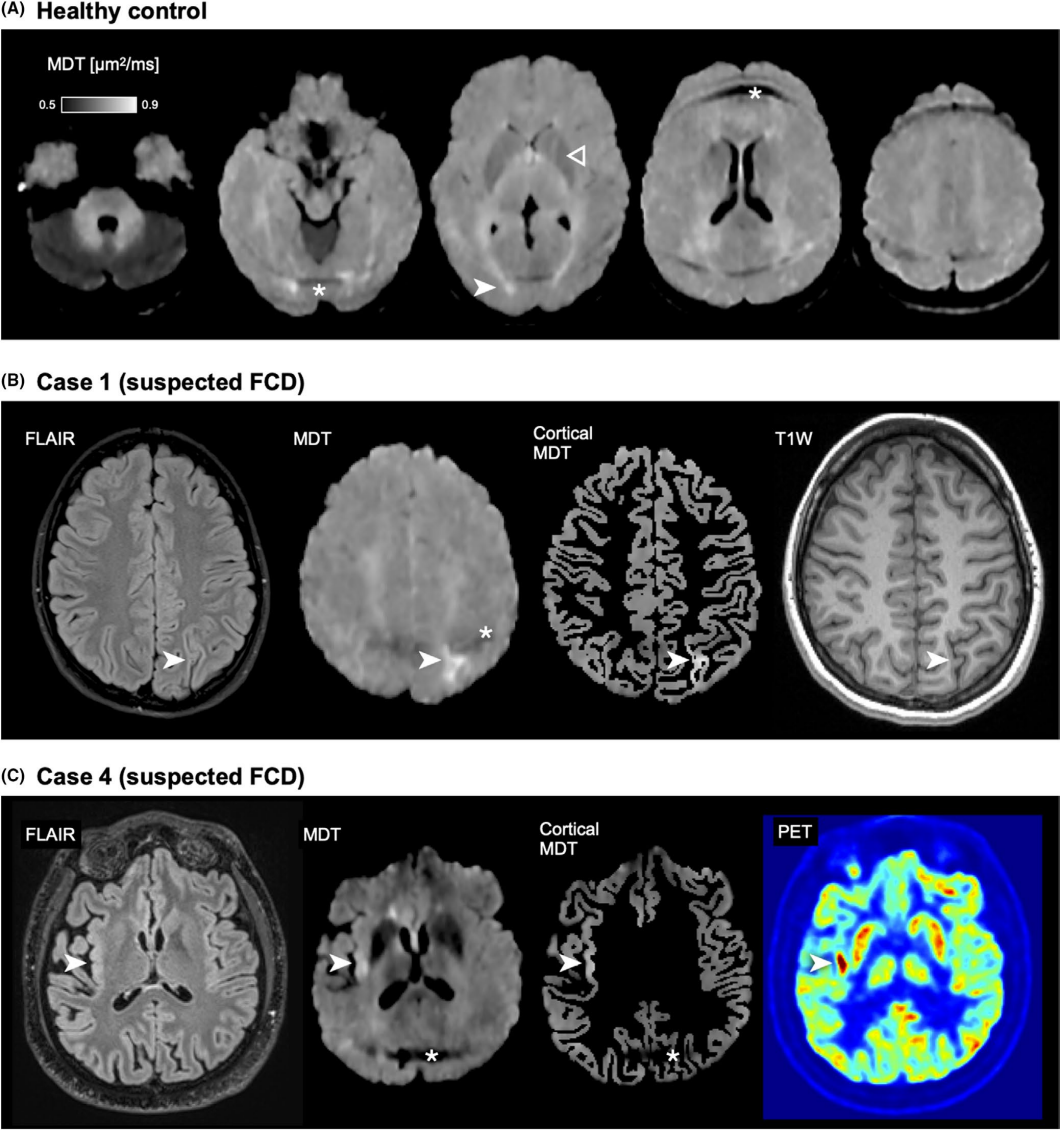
MDT patterns in a healthy participant and suspected focal cortical dysplasias (FCD). Panel A shows characteristic MDT signal features in a healthy brain. Hyperintensities are observed adjacent to the posterior horns coalescing with white matter tracts (white arrowheads), while the basal ganglia appear hypointense (hollow arrowhead). Fat artifacts appear as dark bands, for example, in the cerebellum and supratentorial regions (asterisk). The standard MDT color scale used in this work is shown. Panel B shows a suspected FCD (case 1). The lesion in the left parietal cortex is barely visible on transversal FLAIR and T1W. In contrast, it appeared conspicuous on MDT with standard windowing (white arrowheads). Application of a cortical mask confirmed that the MDT abnormality originates from the cortex. Panel C shows another suspected FCD (case 4). Increased signal on FLAIR and MDT in the right posterior insula (white arrowheads) was colocalized with a hypermetabolic region on PET. Cortical masking again demonstrated that a major component of the MDT intensity is cortical. Asterisks mark fat artifacts (B, C).
